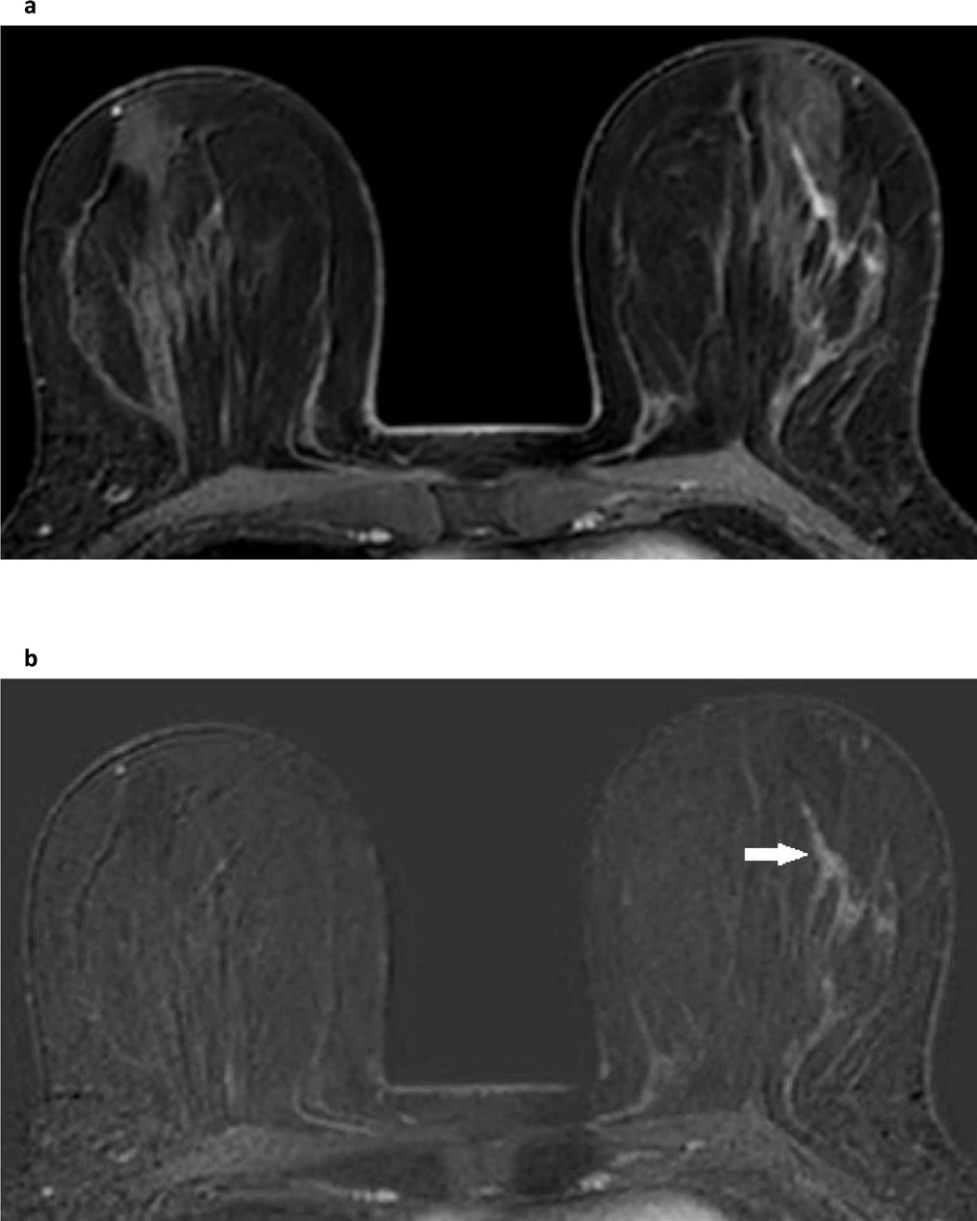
Fibrocystic change as non-mass enhancement. Late post contrast fat-suppressed T1W image from the dynamic series (a) and equivalent subtracted image (b) from the MRI of a high-risk screening client with Li Fraumini syndrome, showing new linear non-mass enhancement (white arrow) in the lateral left breast. MRI-guided biopsy showed that this was fibrocystic change with periductal mastitis.
